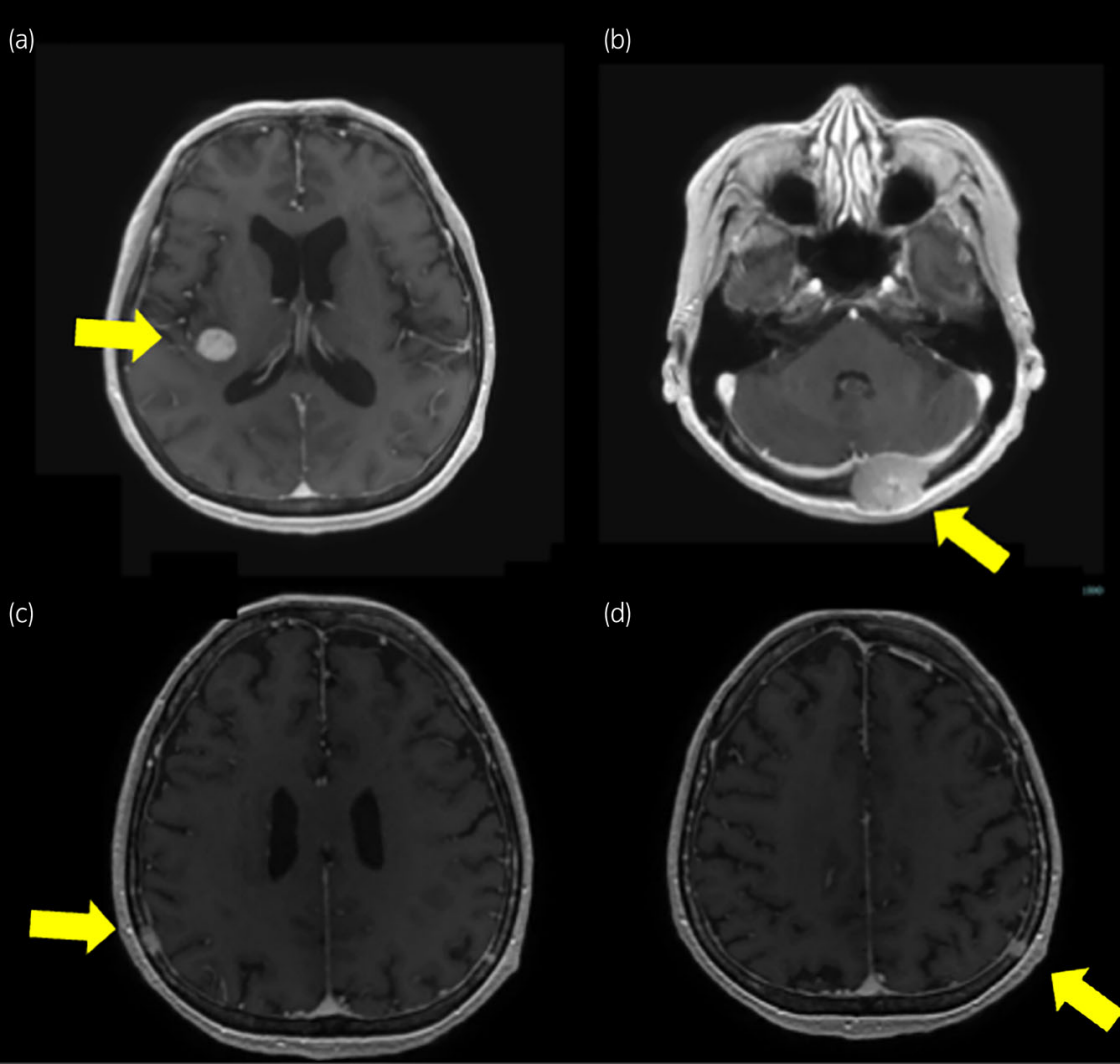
Magnetic resonance images. MR images of the head revealed an intracranial tumor in the right insula (a) and left occipital region with bone destruction (b). In addition, bilateral epidural tumors were observed (c, d). The yellow arrows indicate the positions of the tumors.
